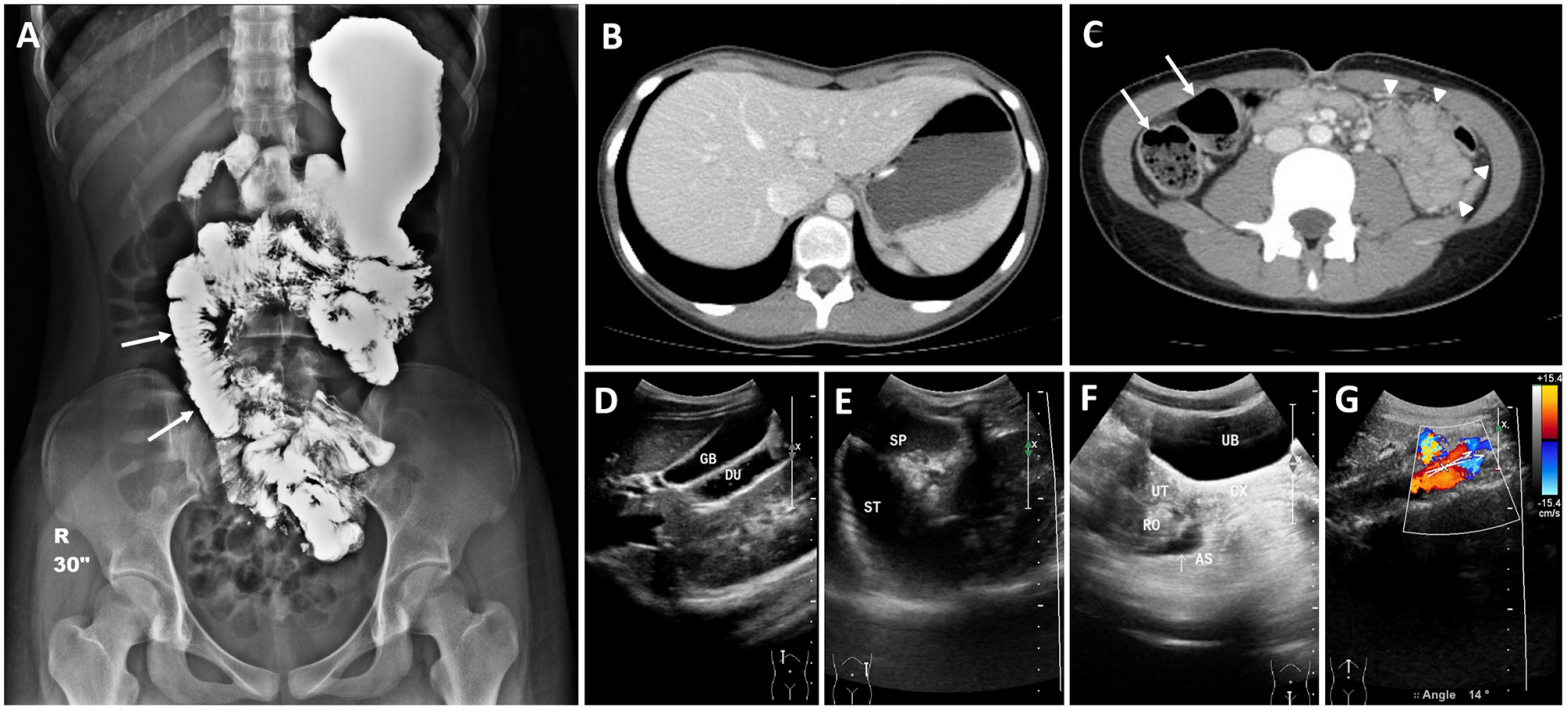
Medical images during third hospitalization of patient with recurrent bilious vomiting. (A) Barium follow-through examination revealed patency in the gastrointestinal tract but segmental dilation at the distal jejunum (arrows), which is compatible with intestinal pseudo-obstruction. CT scan revealed (B) a markedly distended stomach, (C) dilated proximal small intestines with air–fluid levels (arrows) and edematous distal small intestines (arrowheads). Abdominal sonography revealed (D) duodenal dilatation, (E) gastric dilatation, (F) pelvic ascites, and a (G) sharp aortomesenteric angle of 14°. GB, gallbladder; DU, duodenum; SP, spleen; ST, stomach; UB, urinary bladder; UT, uterus; CX, cervix; RO, right ovary; AS, ascites.
